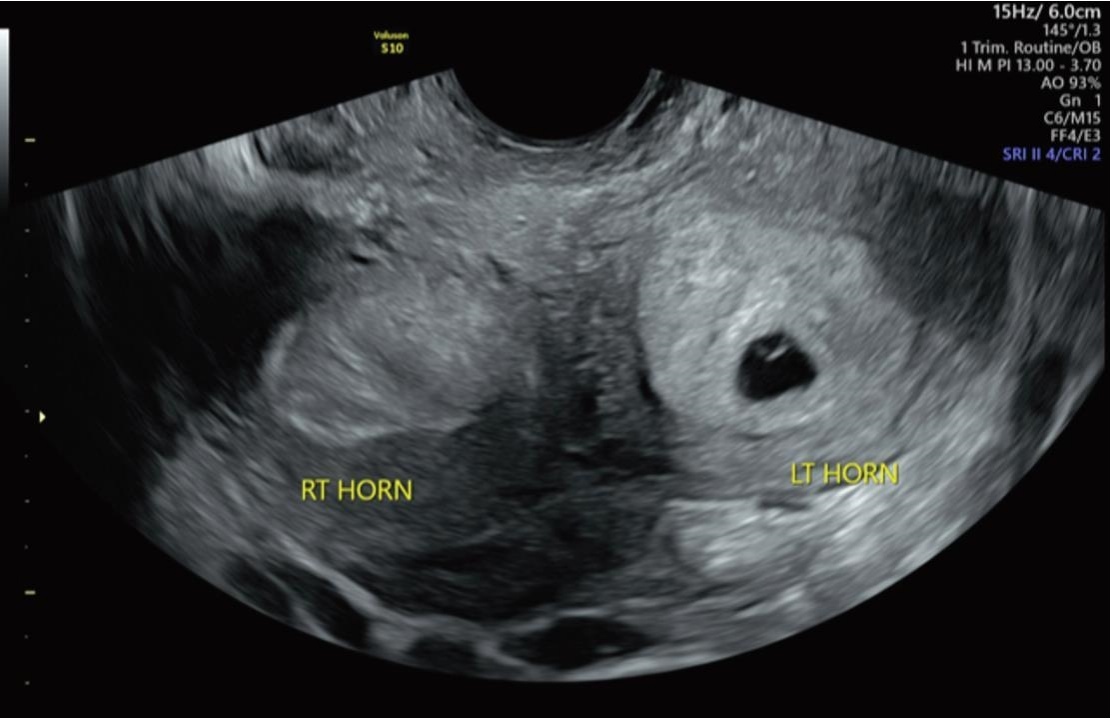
Transvaginal ultrasound showing both uterine horns.
